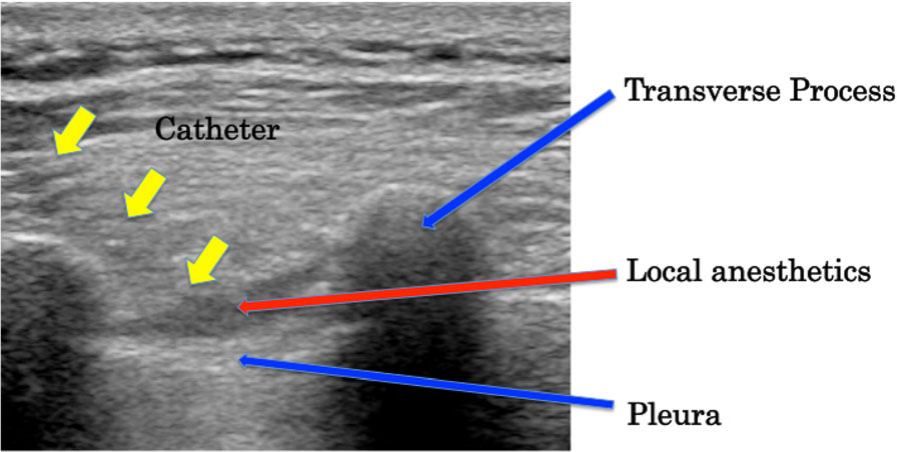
Ultrasound-guided paravertebral block. We confirmed that local anesthetic infused via the catheter spread in paravertebral space
